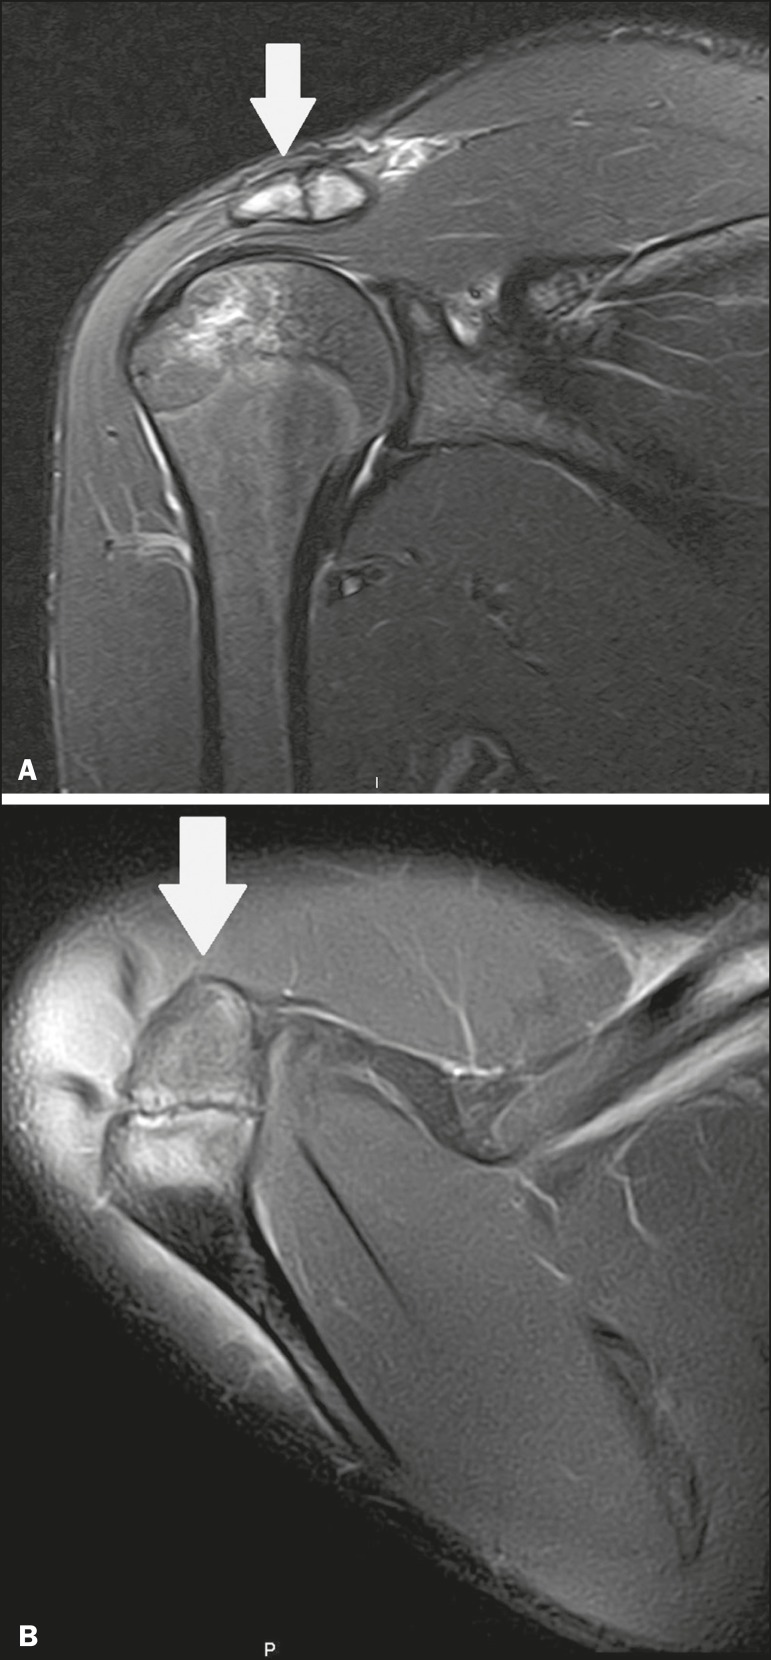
T2-weighted axial and coronal MRI scans of the right shoulder
